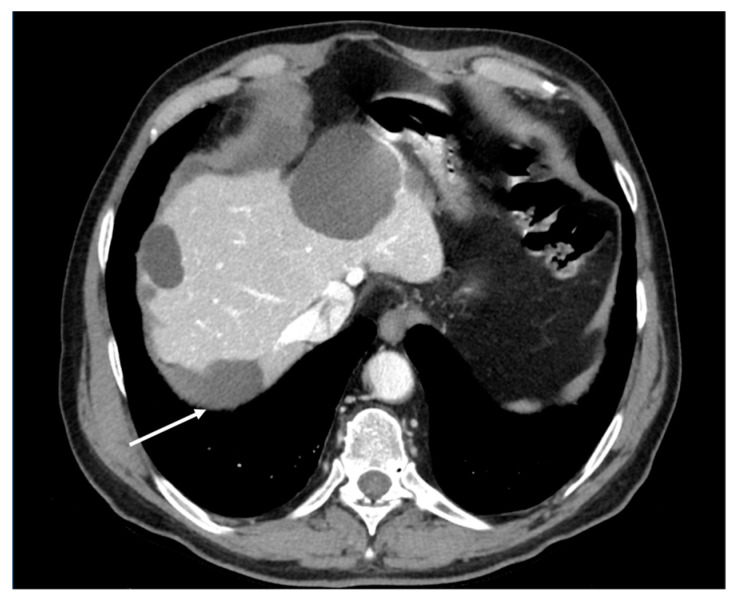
Contrast-enhanced CT scan, axial view. Case of pseudomyxoma peritonei, in a female 63 y. Lesions (white arrow) on the hepatic glissonian surface.
